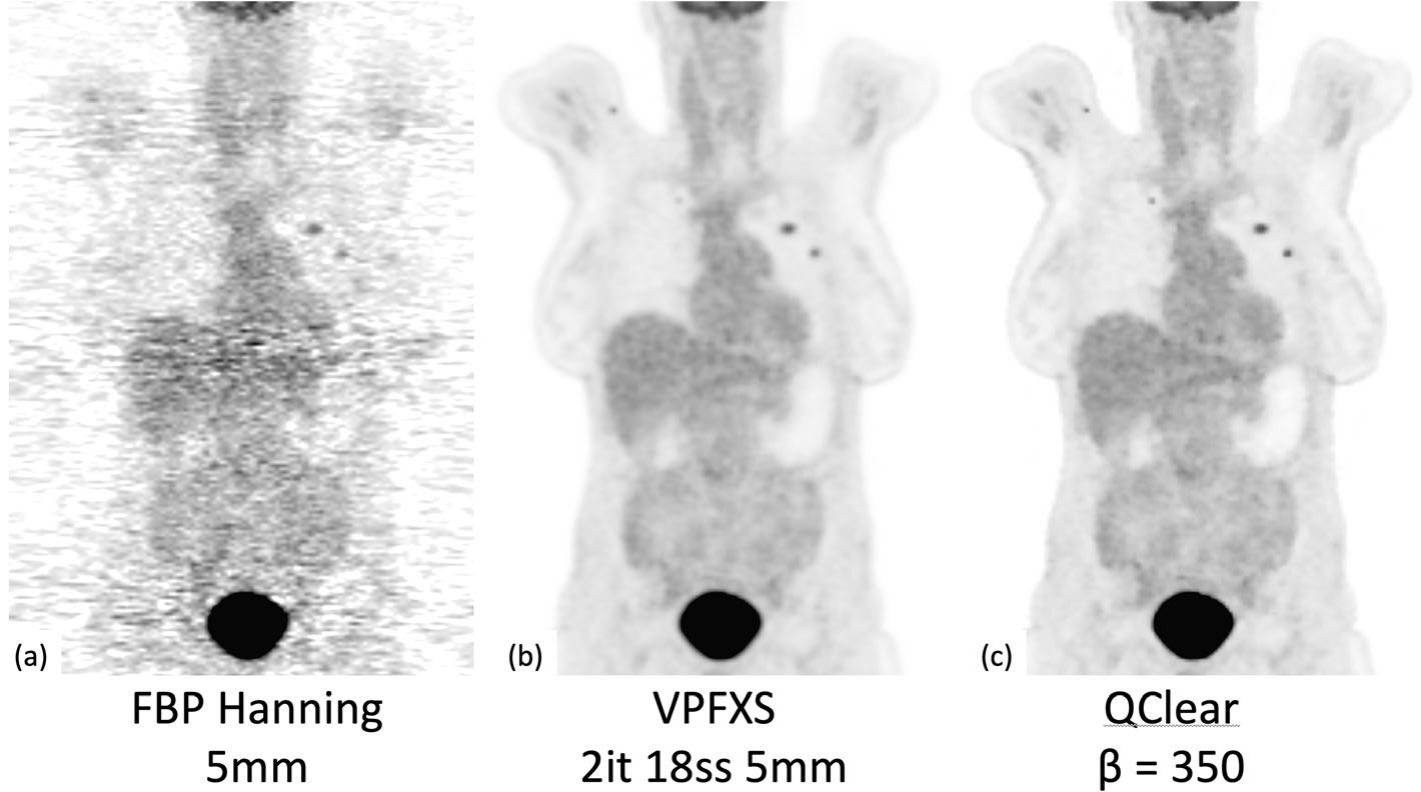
[18F]-FDG PET images of a 71-y/o female (324 MBq, BMI 22) with endometrial adenocarcinoma post hysterectomy, bilateral oophorectomy and lymph node dissection. Images show the differences in quality when using FBP reconstruction (left), iterative reconstruction (middle), and regularized reconstruction (right)
